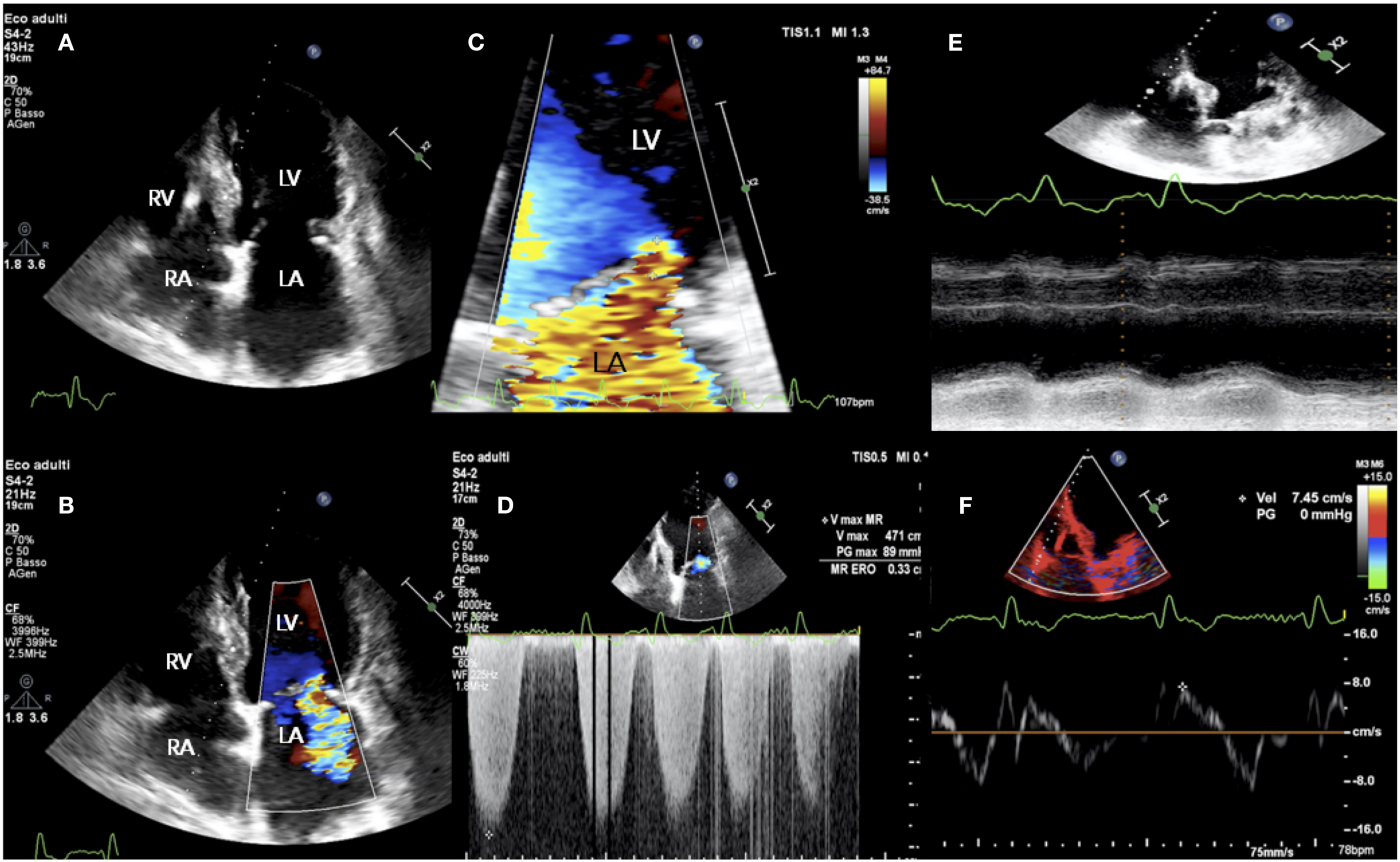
TTE study in a patient presenting with acute signs and symptoms of HF, NYHA class III, NT-proBNP 6,459 pg/ml, and “disproportionate” functional MR. (A) LV end-diastolic volume of 175 ml; (B–D) holosystolic, central jet of MR and EROA of 0.34 cm^2^; (E,F) RV longitudinal dysfunction with TAPSE 10 mm and S' 7 cm/sec. EROA, effective regurgitant orifice area; HF, heart failure; NYHA, New York Heart Association; NTproBNP, N-Terminal pro-B-type natriuretic peptide; TAPSE, tricuspid annular plane systolic excursion; TR, tricuspid regurgitation; TRV, TR velocity; TTE, transthoracic echocardiography; other abbreviations as in previous figures.
